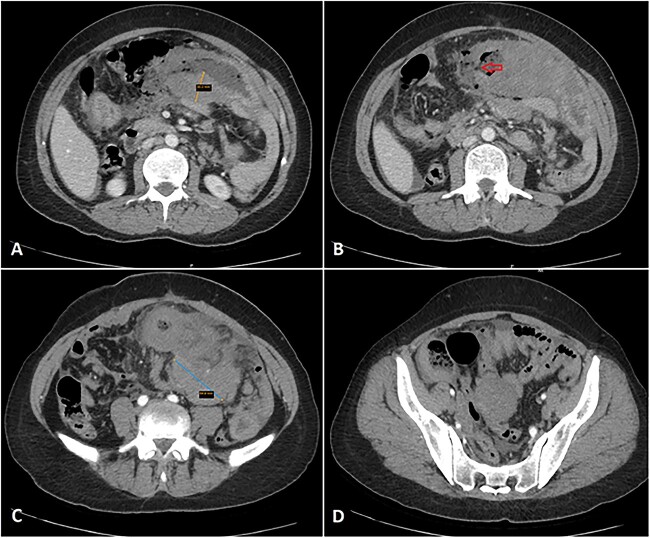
Multiple axial cuts of the patient’s abdomen CT scan with IV contrast are shown. Extensive bowel wall thickening up to 3 cm is noted, involving a long segment of small bowel loops in the left abdomen (jejunal loops) with a gray pattern of enhancement (A). Additionally, a wall defect/perforation is seen with the surrounding pneumoperitoneum and spillage of fecal material within the peritoneal cavity (B). Associated multiple enlarged adjacent mesenteric lymph nodes are also demonstrated, forming a large mass-like lesion with surrounding fat stranding (C). Moreover, mild wall thickening involving another short segment of small bowel loops in the right abdomen (ileal loops) is also noted (D).
